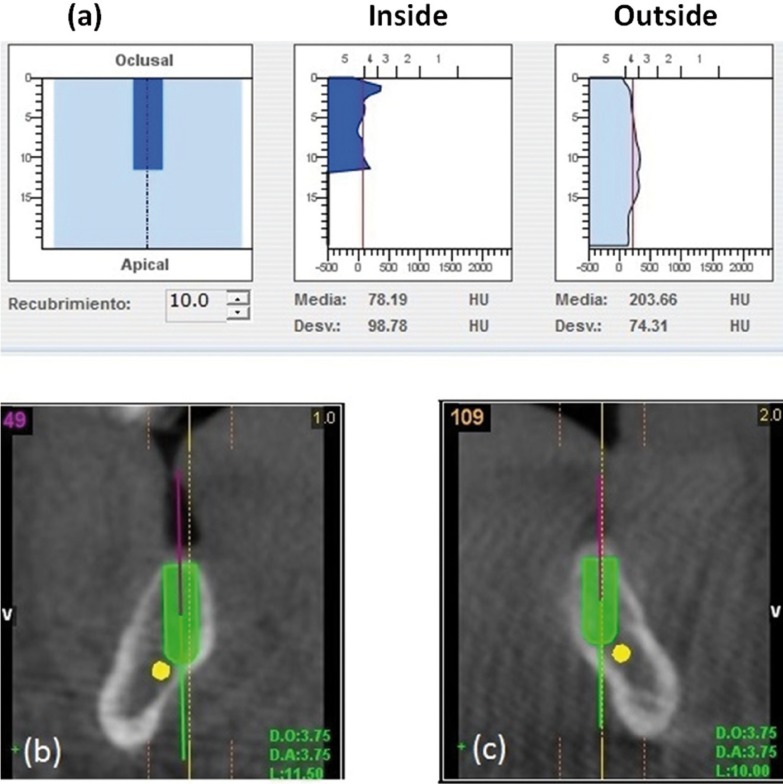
CBCT (a) Bone density in cancellous bone. (b) Planning implant 46. Bicortical anchorage. (c) Planning implant 36. Bicortical anchorage.
